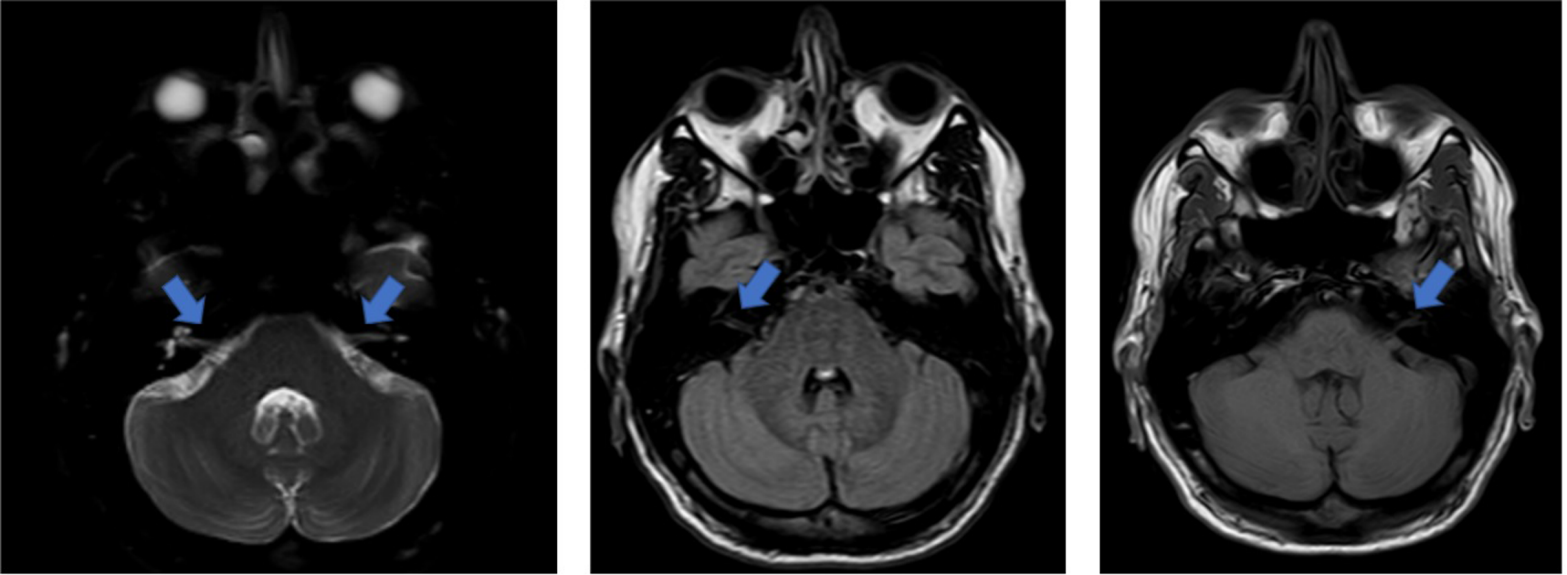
Normal VII‐VIII nerves in brain MRI.
